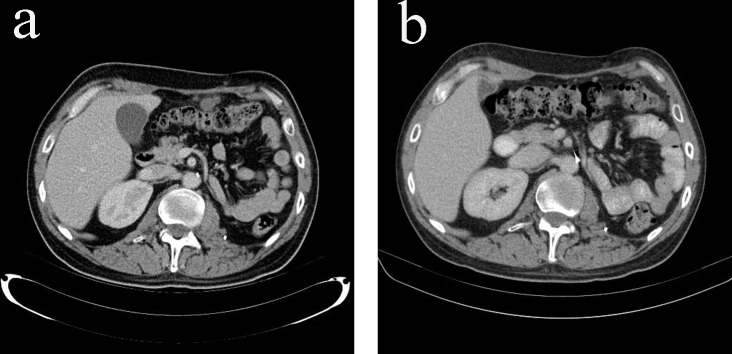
(a) Peritoneal metastases in CT scan (transverse view) before additional chemotherapy with EDP. (b) Resolution of peritoneal metastases in CT scan (transverse view) after additional chemotherapy with EDP.
